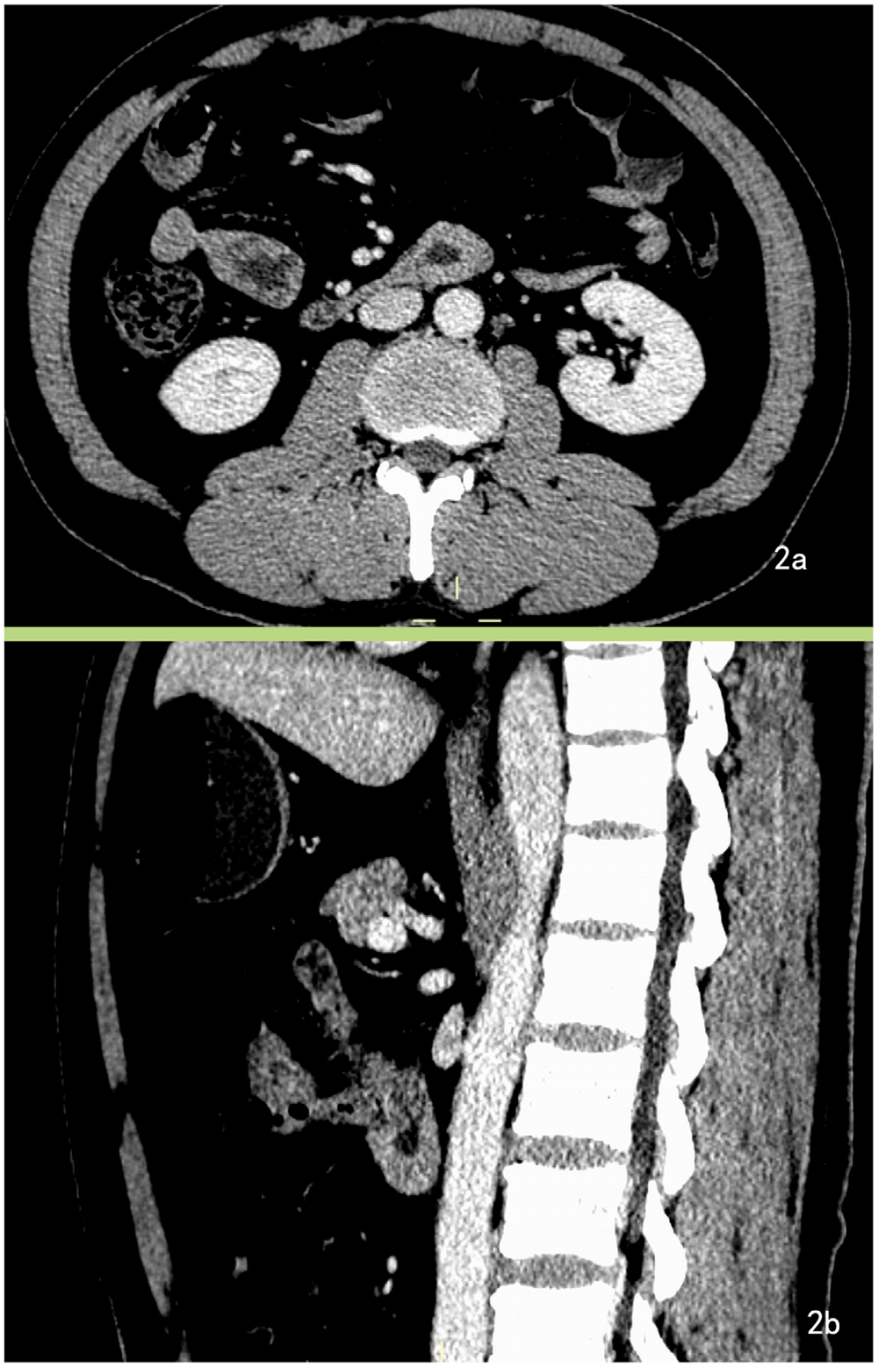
GIST overlooked in CT images. A 64-year-old male with duodenal GISTs presented with melena for the past 20 days. A. Enhanced CT image demonstrates bowel wall thickening at the third segment on the axial section of the CT image and was overlooked. B. Post-procedural CT images (sagittal section) reveal the lesion more clearly. One radiologist had overlooked the lesion, while the other radiologist had misdiagnosed it as a heterotopic pancreas.
